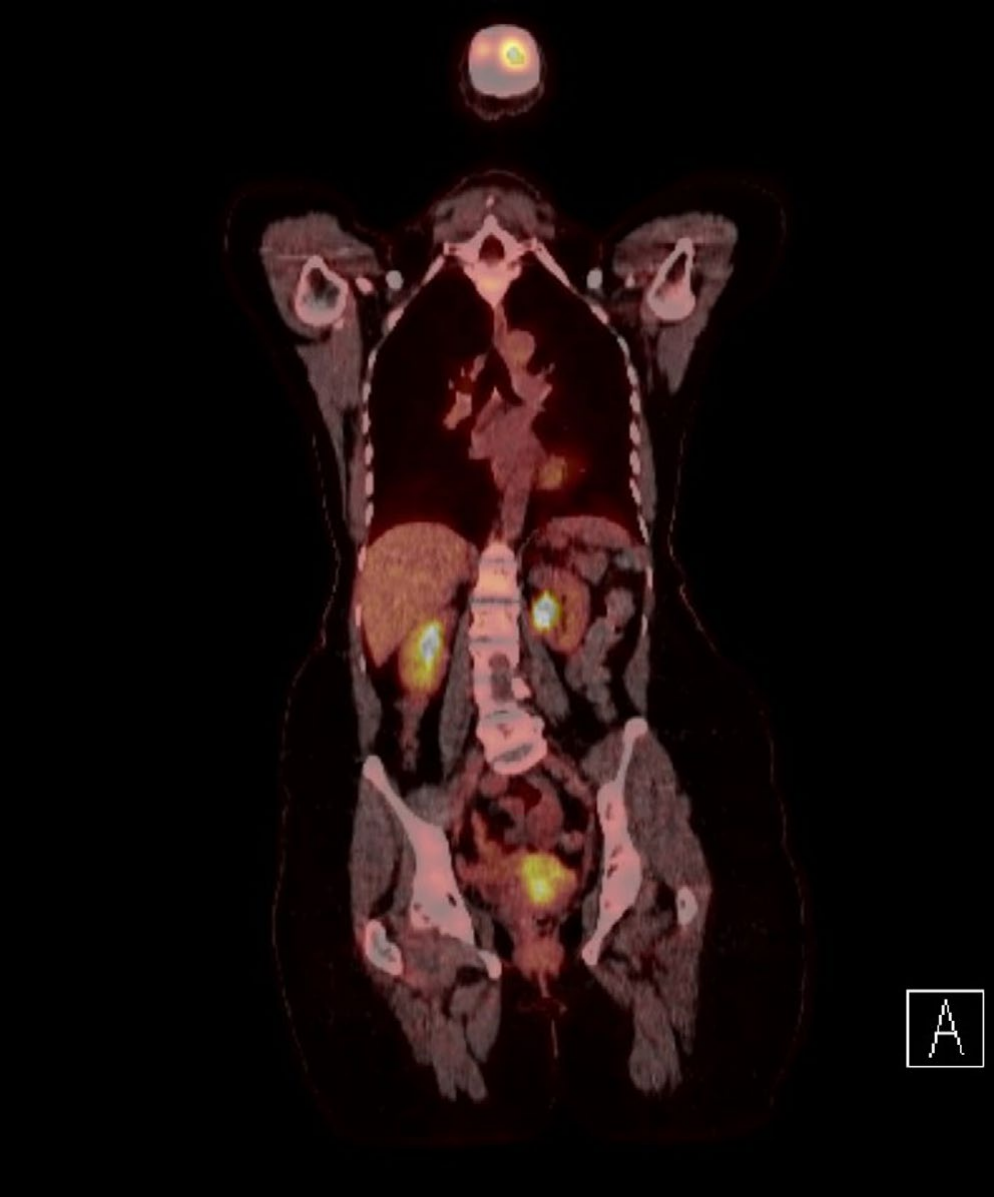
PET scan (prior to completion of therapy) showed partial metabolic response to treatment with significant decrease in extent and avidity of cervical lesion (SUV max 8.1, previously 13.5). No evidence of metabolically active local nodal or distant metastatic disease
